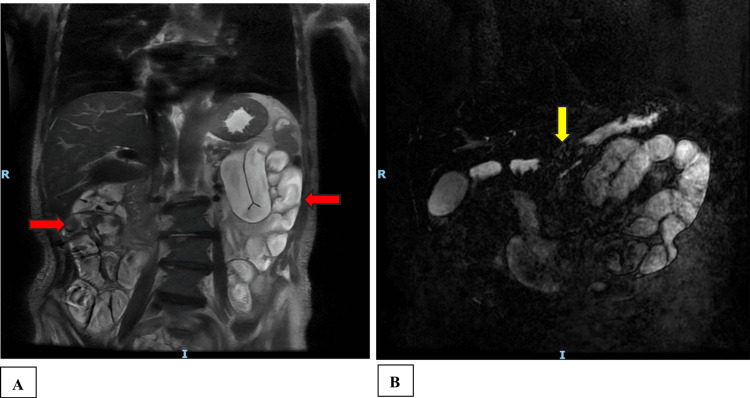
MRI abdomen and pelvis with intravenous contrast In A, there is mildly dilated, fluid-filled small bowel loops throughout the abdomen marked by the red arrows and in B there is evidence of decreased T1 signal throughout the pancreatic body marked by the yellow arrow.
